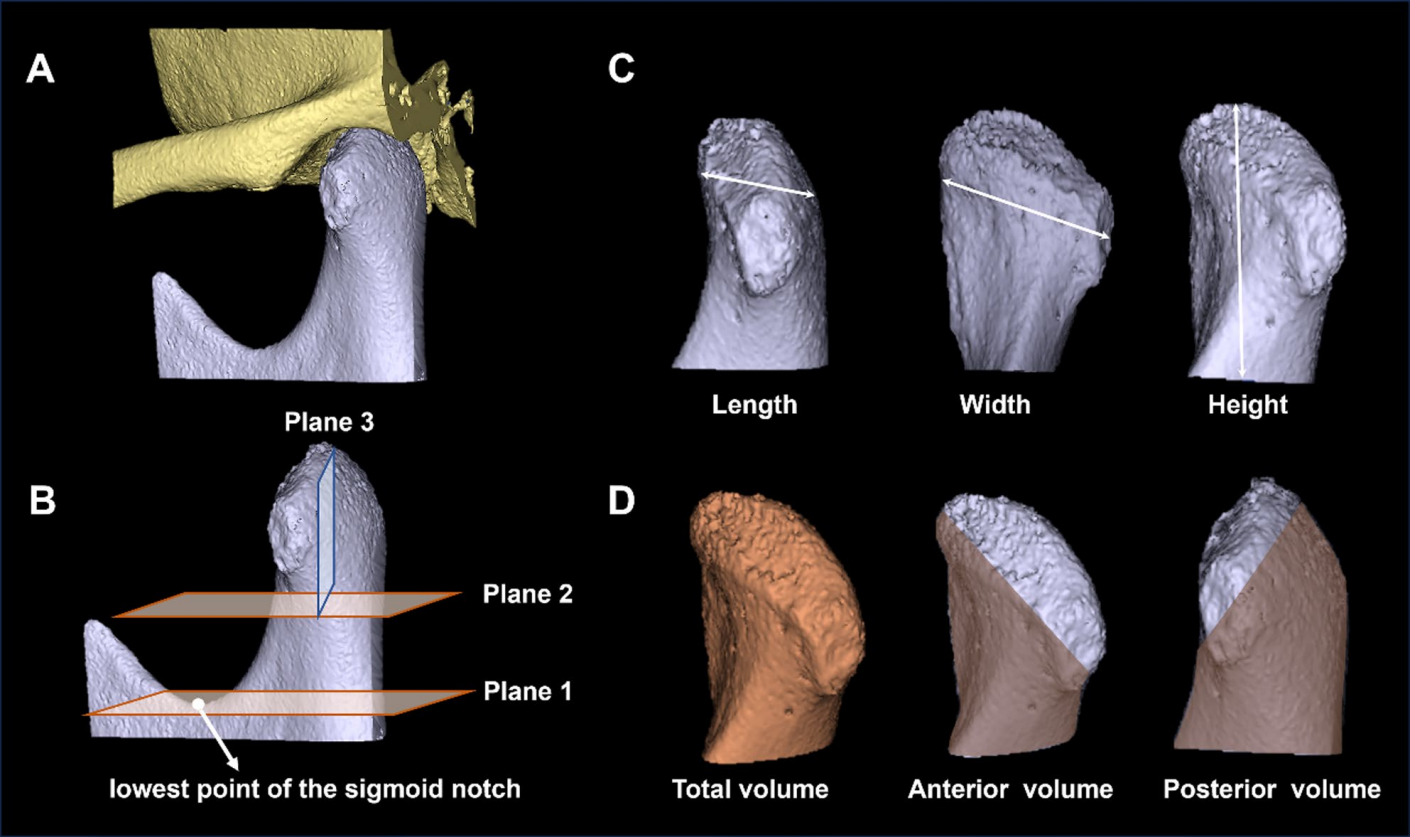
Measurement of condylar morphology on U-HRCT -based 3D models (A). B Standard observation planes. Plane 1 is the horizontal plane passing through the lowest point of the sigmoid notch, Plane 2 is positioned mid-height of the condyle, and Plane 3 is the coronal plane passing through the condylar apex. C measurement of condylar length, width, and height. D measurement of total, anterior, and posterior volumes (orange)
